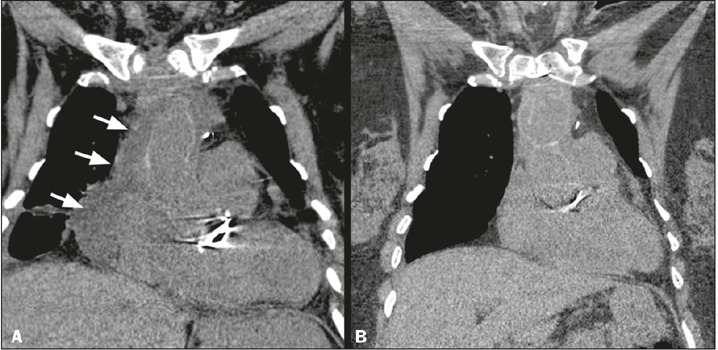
Normal postoperative sternotomy findings (coronal CT images; mediastinal
